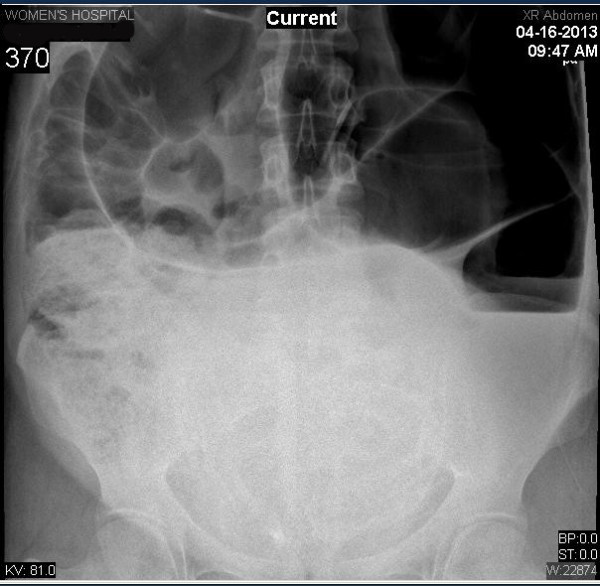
X-ray examination showing the enormous dilatation and complete occlusion of the sigmoid.
